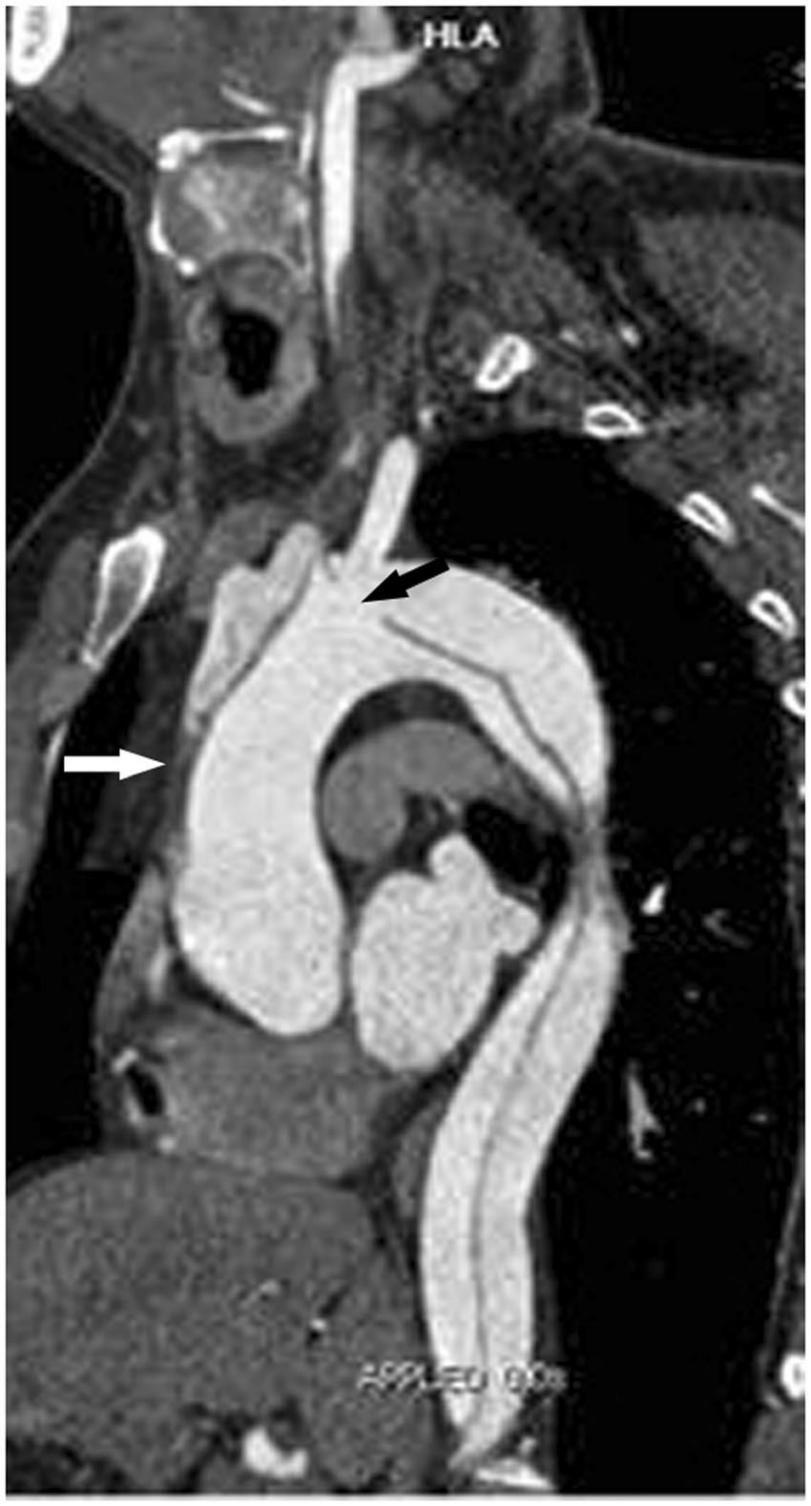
Two CTA Risk Findings for Postoperative PND. Oblique sagittal MPR image showing dissection involving the thoracic aorta and supra-aortic branches. An entry tear (black arrow) is located in the aortic arch, and the intimal flap extends into the aAO in a retrograde fashion. Note the thrombosis (white arrow) in the false lumen, due to the integrated intimal flap in the aAO. The two CTA findings, which exist simultaneously, indicate an extremely high risk of thrombus in the false lumen extending to the cerebrum via the entry point. CTA = CT angiography; PND = permanent neurological dysfunction; MPR = multiplanar reformation; aAO = ascending aorta.
